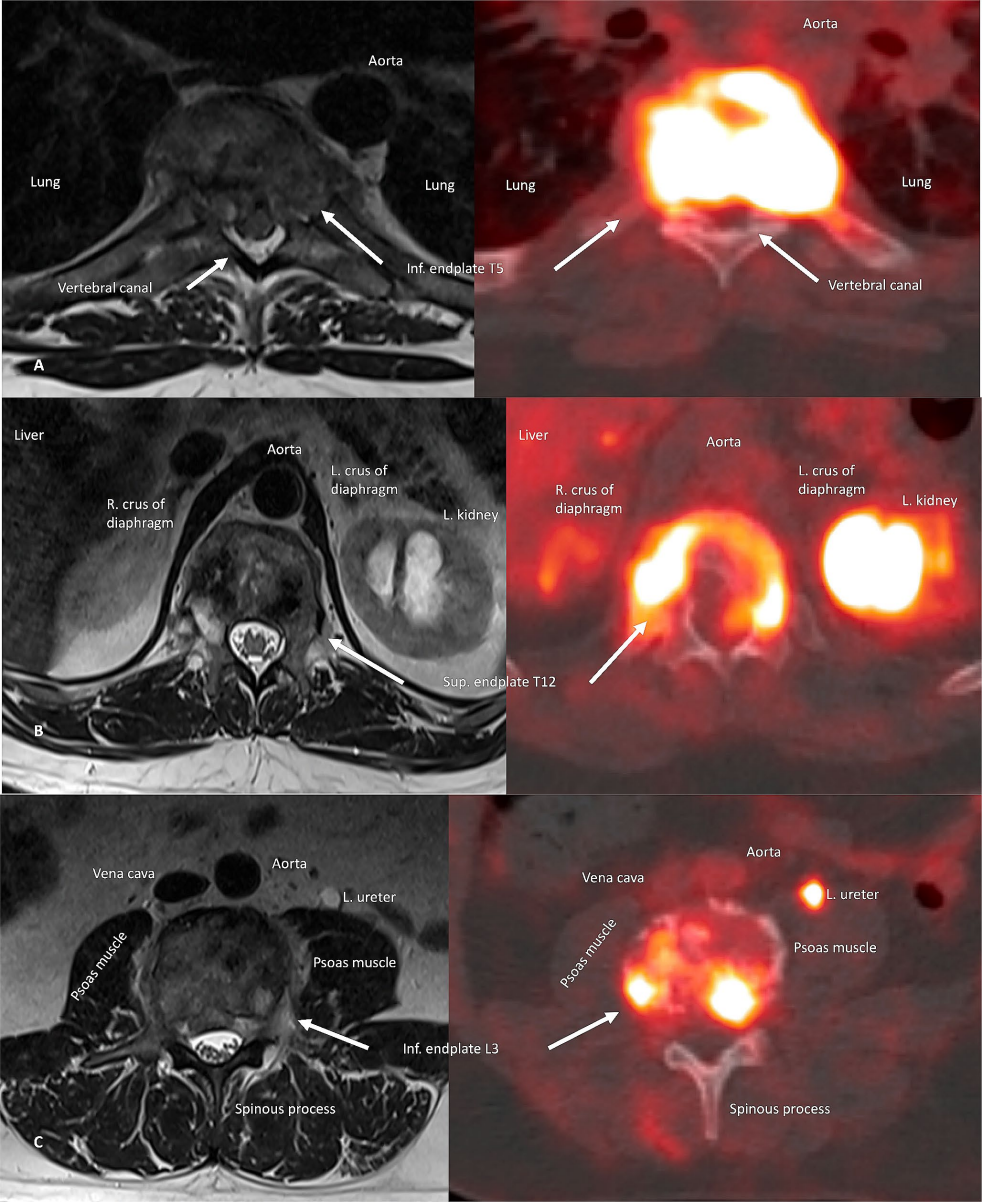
A: T5/6 lesion on MRI and 18-FDG-PET/CT on axial plane. B: T11/12 lesion on MRI and 18-FDG-PET/CT on axial plane. C: L3/4 lesion on MRI and 18-FDG-PET/CT on axial plane
